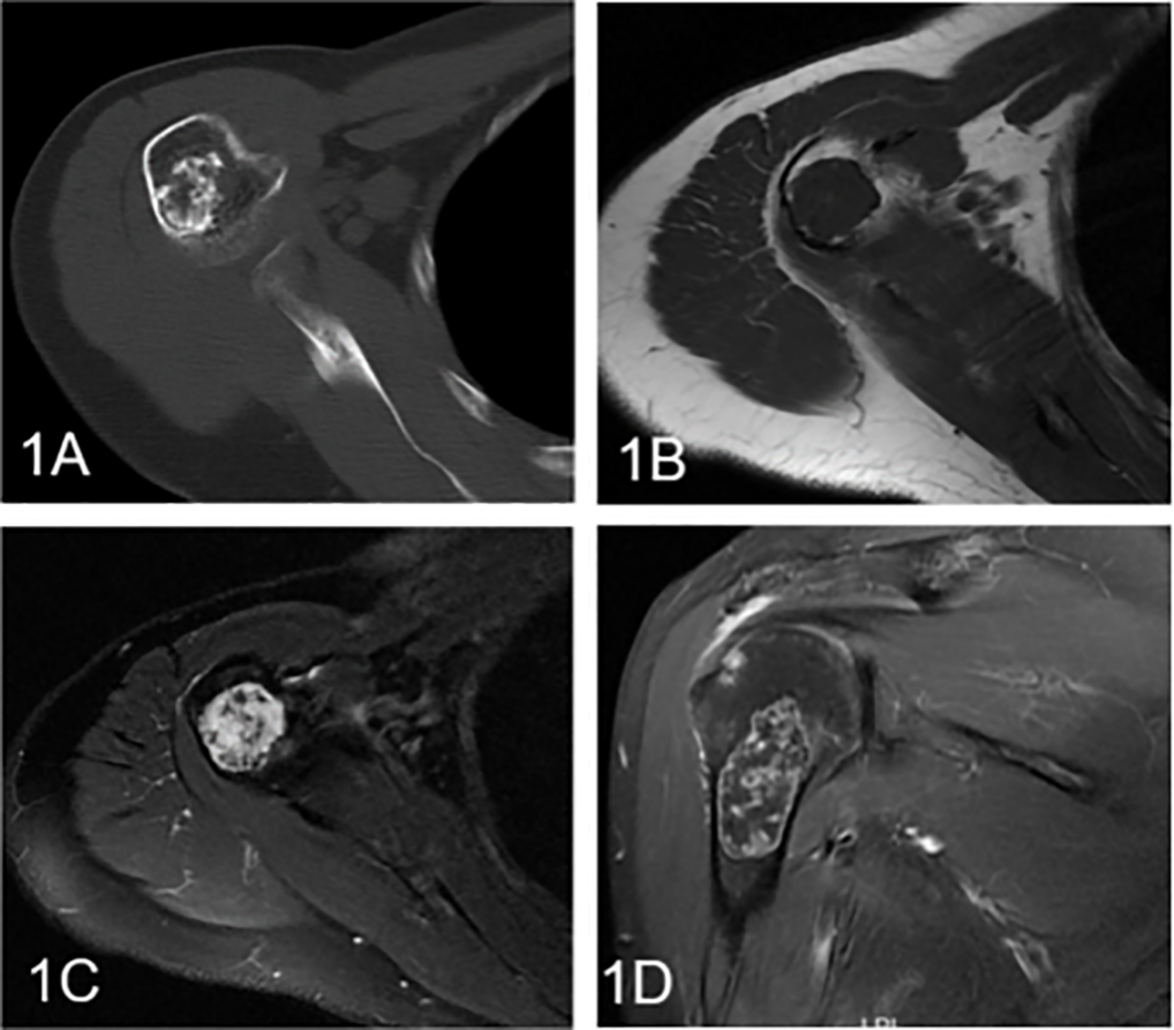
Enchondroma involving the humerus in a 58-year-old female. (A) Axial CT shows a well-defined intramedullary osteogenic lesion in the metaphysis of the right humerus, which presents with characteristic chondroid (ring and arc) calcifications. (B) Axial T1WI presents a mass with global heterogeneity and a well-defined margin. (C) Axial fat-suppressed T2WI shows a heterogeneous hyperintense mass with low signal foci. (D) Coronal fat-suppressed contrast-enhanced T1WI demonstrates marginal and septal or ring-and-arc enhancement within the lesion.
